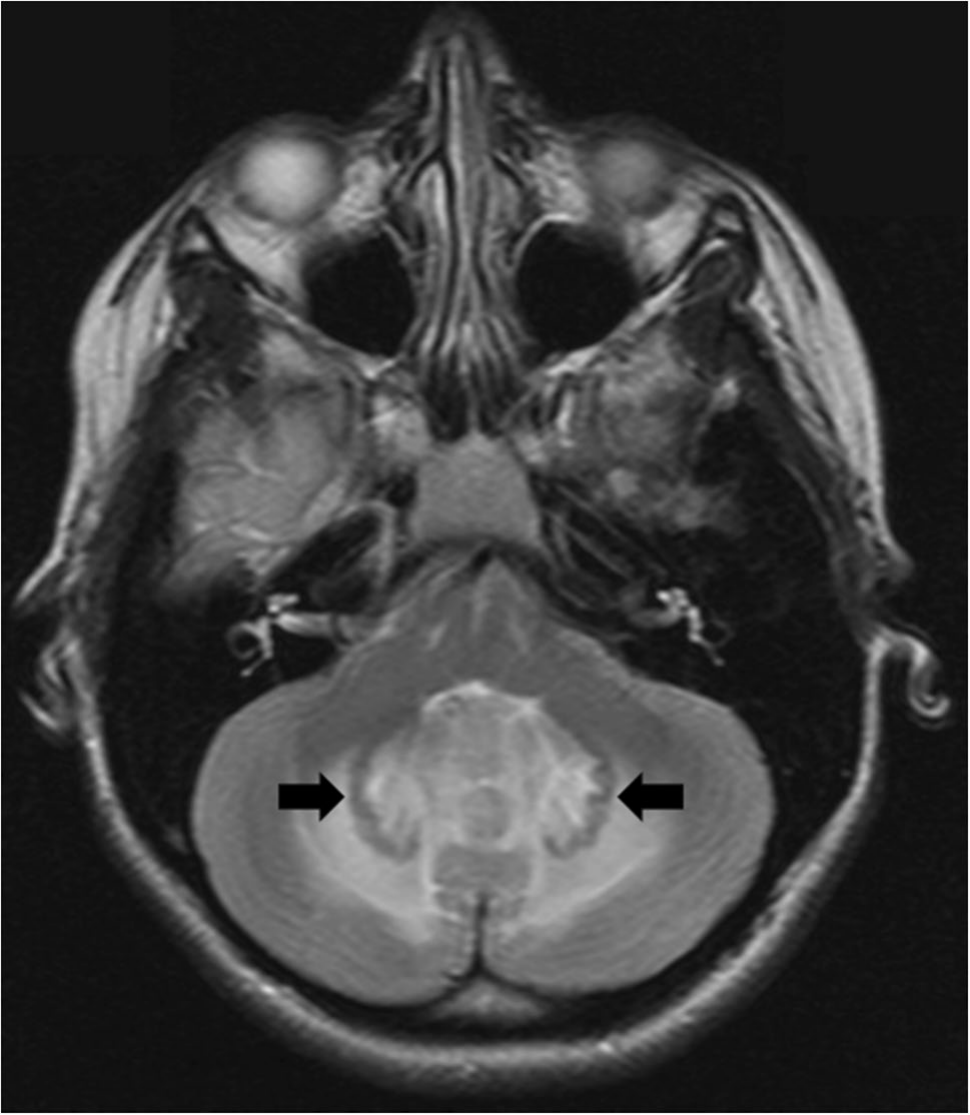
Axial T2-weighted sequence of a preoperative MRI showing the DN on both sides (arrows) within the perifocal edema surrounding a midline cerebellar tumor
